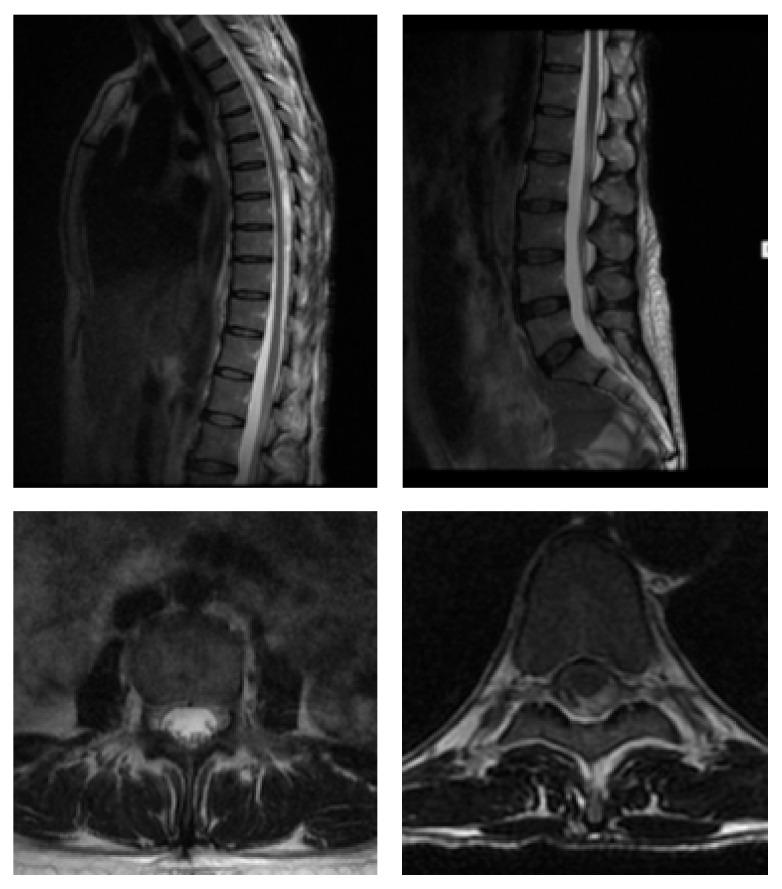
Sagittal and axial T2 weighted MRI images. Vertebral height, disc height, alignment, and bone marrow signal are within normal limits. The canal and neural exit foramina are capacious at all levels.
